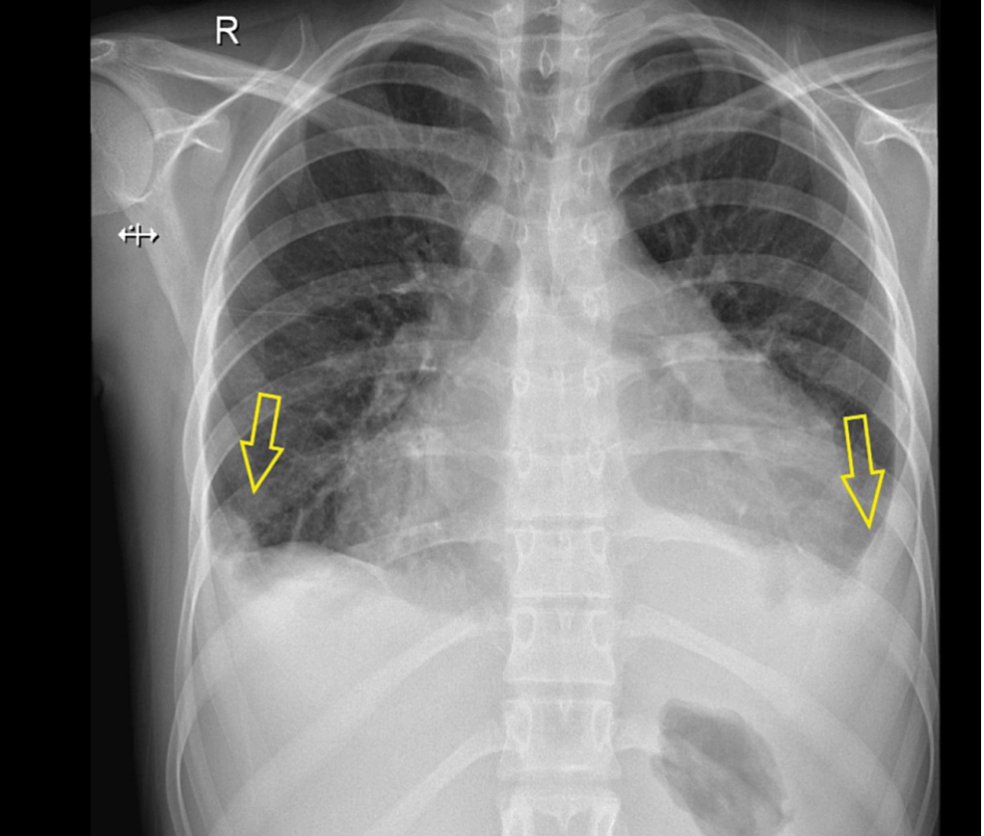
Initial chest imaging before initiating the management plan Image description: posterior-anterior view of the erect chest X-ray showing cardiomegaly with a silhouette sign and bilateral obliteration of costophrenic angle more in the left side, which goes with bilateral pleural effusion.
